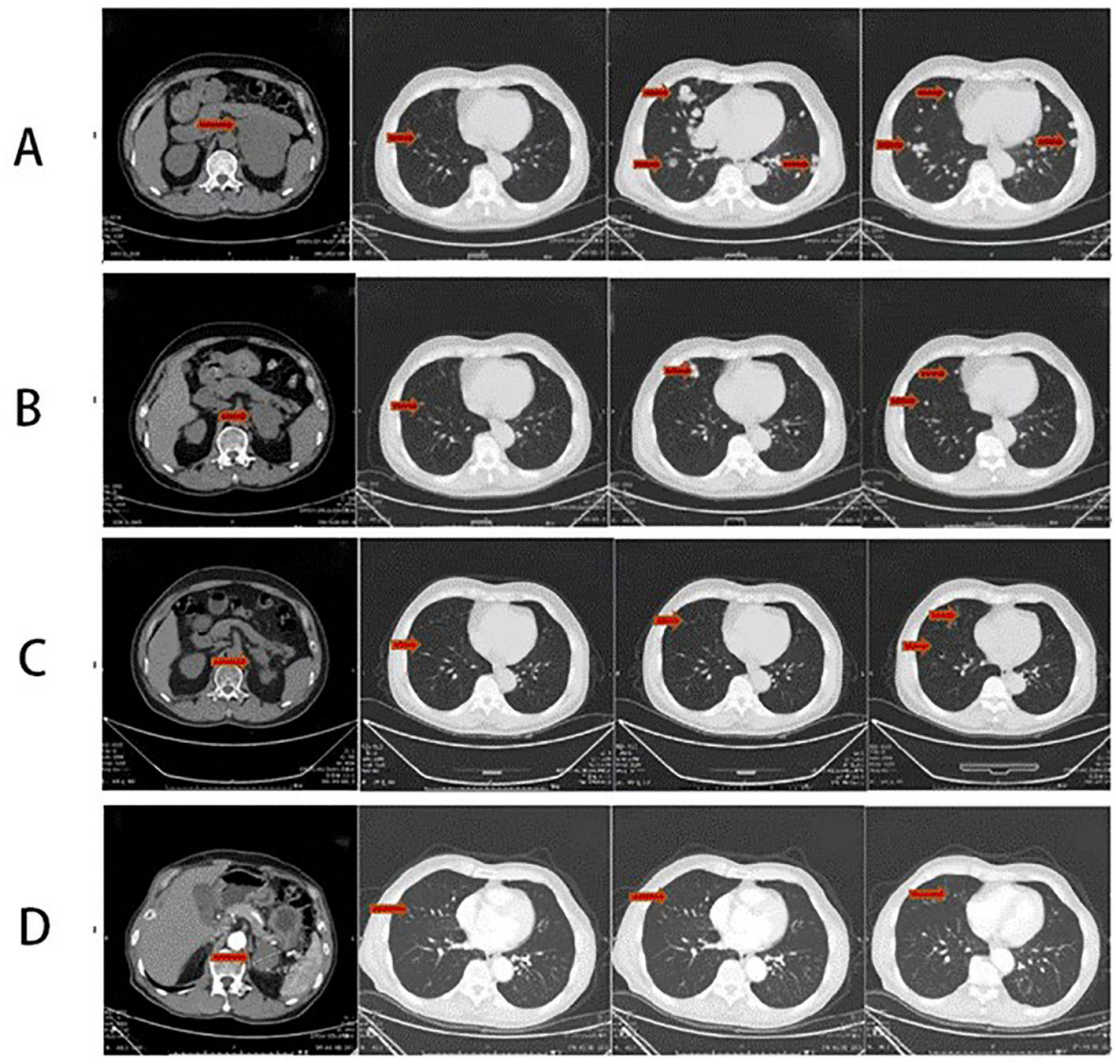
(A) Thoracic and abdominal CT scan before the therapy (2020-08) showing the following: mass in the left adrenal gland measuring approximately 110 mm × 87 mm suggestive of malignant tumor and multiple nodules in bilateral lungs suggestive of metastasis. (B) Thoracic and abdominal CT scan after four-cycle EP chemotherapy and anlotinib combined with sintilimab immunotherapy (2021-3): the left adrenal mass has significantly subsided, with a size of approximately 51 mm × 67 mm. Reduction in bilateral multiple pulmonary metastatic nodules. (C) Thoracic and abdominal CT scan after maintenance therapy for 8 months (2021-11): a mass in the left adrenal gland area with a cross-section of approximately 41 mm × 28 mm. The size of multiple bilateral lung metastasis further decreased. (D) Thoracic and abdominal CT scan after maintenance therapy for 24 months (2023-03): a mass in the left adrenal gland area with a cross-section of approximately 39 mm × 29 mm. The size of multiple bilateral lung metastasis further decreased. The whole red arrowheads show the before and after treatment of primary lesion of the left adrenal gland and the metastatic lesions of the lungs.
